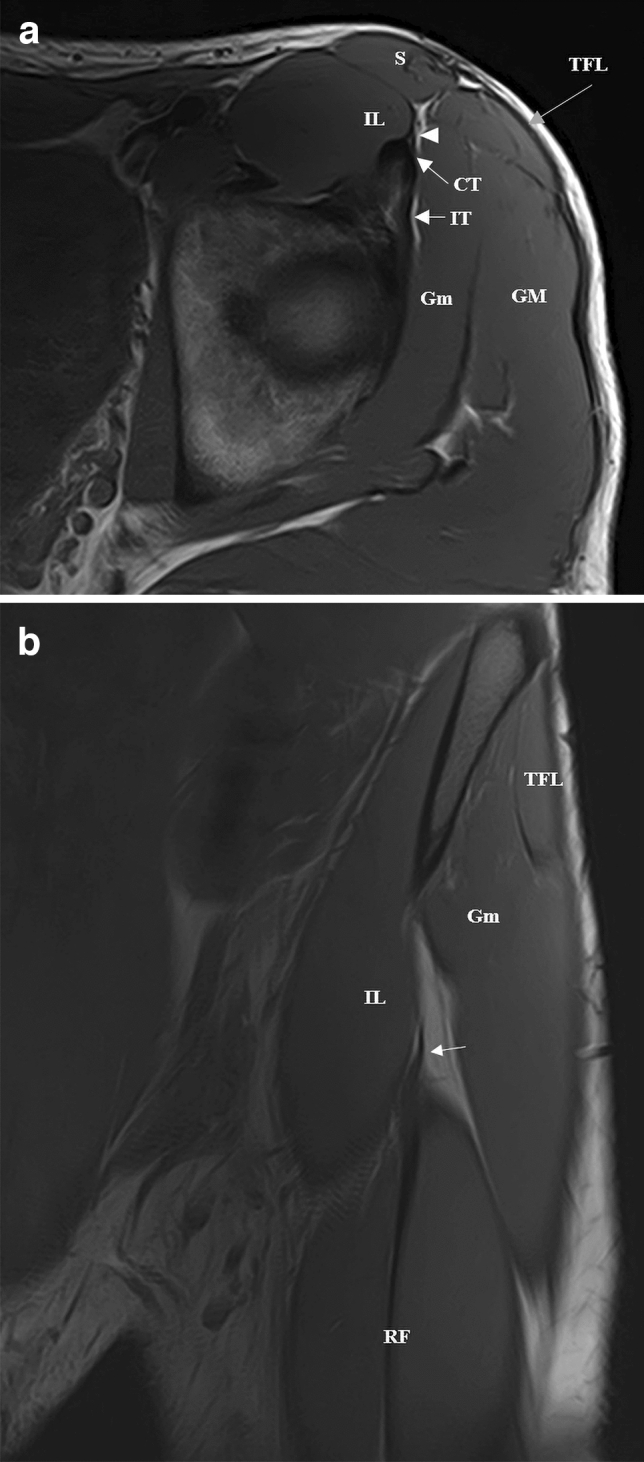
a Axial T1 weighted magnetic resonance (MR) image of the left pelvis. We can see the membrane (arrowhead) a thin hypointense band lateral to the iliac muscle (IL) and its relation with the proximal portion of the CT. IT; S; TFL; gluteus minimus (Gm); gluteus magnus (GM). b Coronal T1 weighted MR image of the left pelvis. We can see a thicker hypointense membrane (arrow) lateral to the IL and its distal insertion to the distal portion of the CT. Its heterogeneous signal could be secondary to the presence of fat tissue within the connective tissue. RF; S; TFL; Gm
